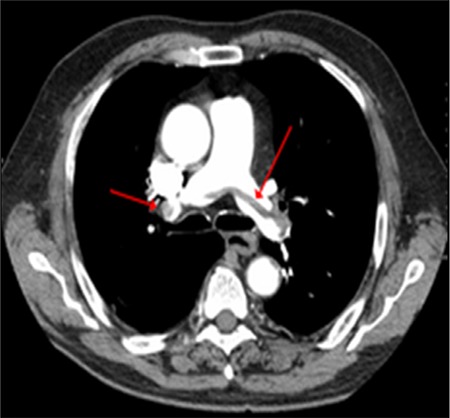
Thorax computed tomography angiography of the patient demonstrating filling defects in right pulmonary artery (arrows).
